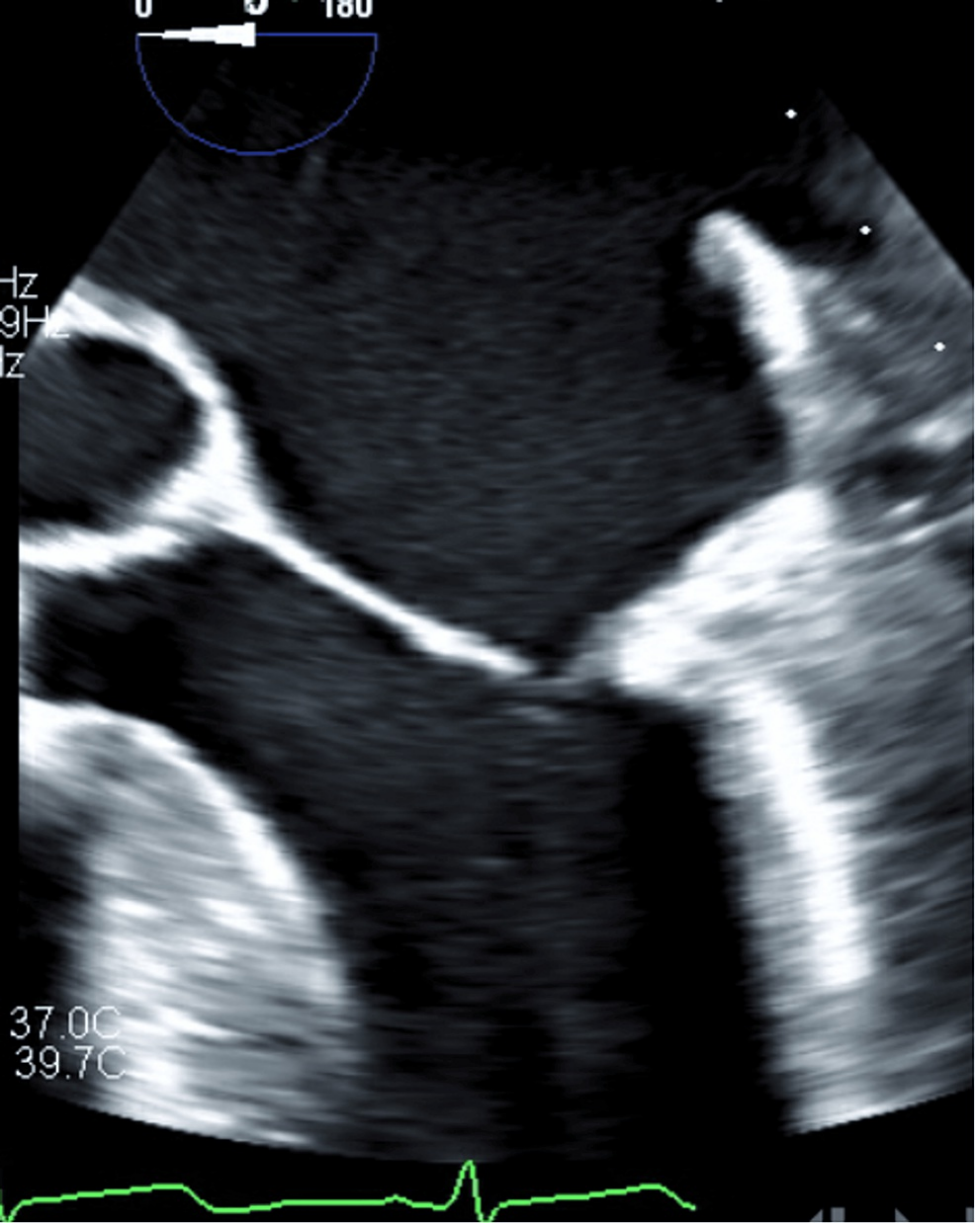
Transesophageal echocardiogram post procedure showing complete vegetation removal
